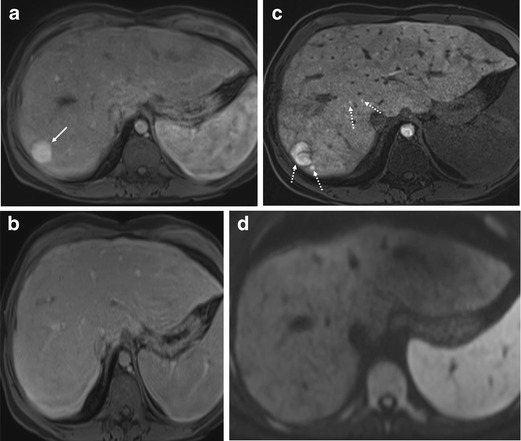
Focal nodular hyperplasia-like nodules in post-Fontan patients. a-d A 21-year-old woman with previous double inlet left ventricle. a There is an approximately 2.3 cm homogeneous hypervascular nodule in segment 7, periphery of the liver (*arrow*) on the axial arterial phase post contrast T1-weighted MR image. b The lesion showed iso-signal intensity with no hypointensity on the axial transitional phase post contrast T1-weighted MR image. c The lesion with a few other small foci (*dotted arrows*) showing increased contrast material uptake in the hepatobiliary phase T1-weighted MR image with gadoxetate disodium. d Single-shot echo-planar diffusion-weighted image (*b* = 1,000 s/mm^2^) demonstrates no diffusion restriction in the corresponding lesion
